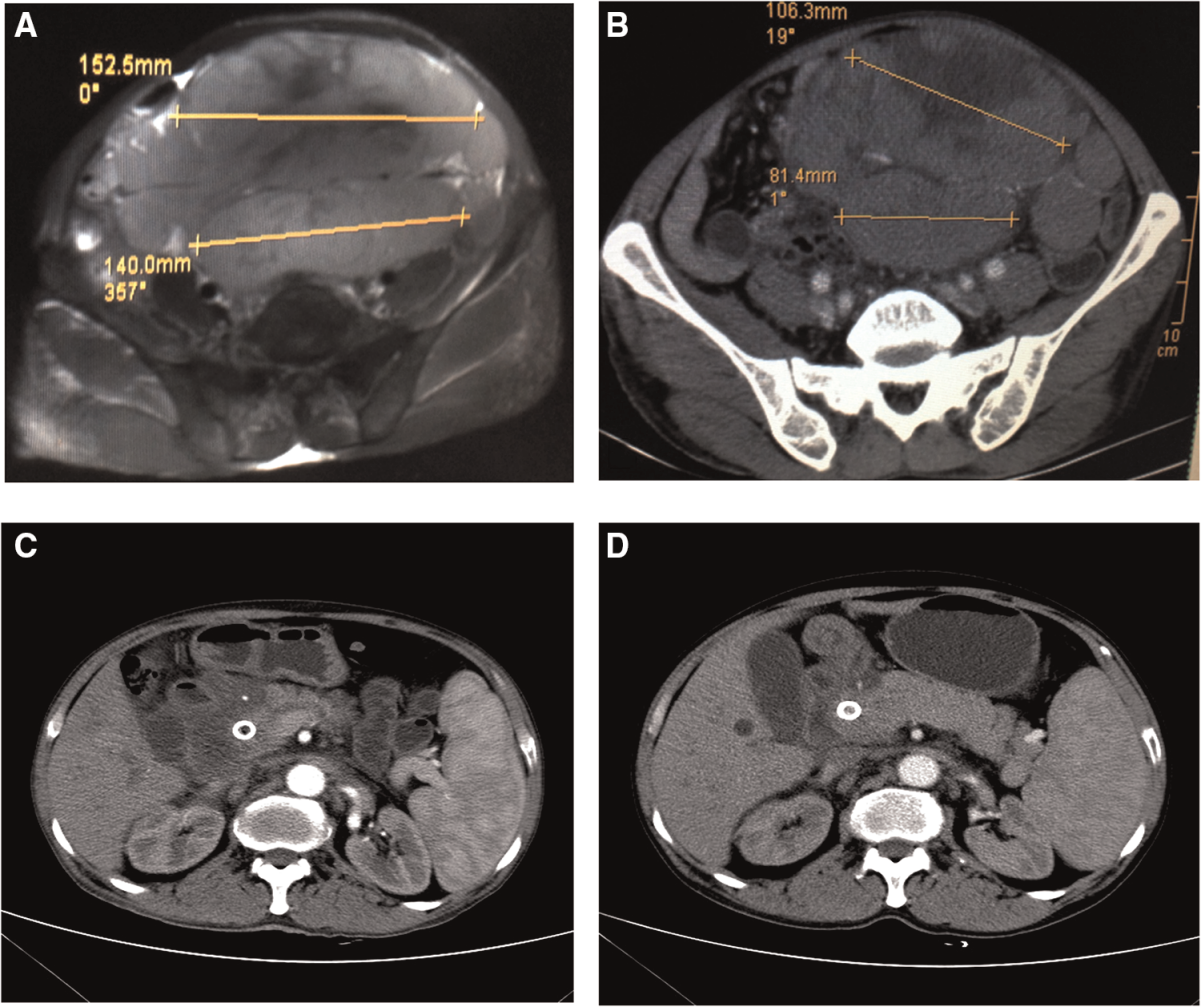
Computed tomography (CT) findings before and after endoscopic ultrasonography–guided biliary drainage. (A) A CT image obtained shows a large lump in the abdominal cavity. (B) An image obtained (one month after EUS-BD) shows significant shrinking of the abdominal mass. (C) CT re-examination (four month after EUS-BD) showed that most of the mass shadows in the abdomen, pelvic cavity, and retroperitoneal cavity were significantly smaller than previously, the pressure on the abdominal viscera was reduced, and the expansion of the portal vein trunk and left and right branches was lessening, with no apparent change in the common bile duct. (D) A review total abdominal CT (one year later) showed that abdominal and retroperitoneal lymph node enlargement, little change in internal and external bile duct dilatation,more absorption of gas in the biliary system than previously, and common bile duct stent implantation.
